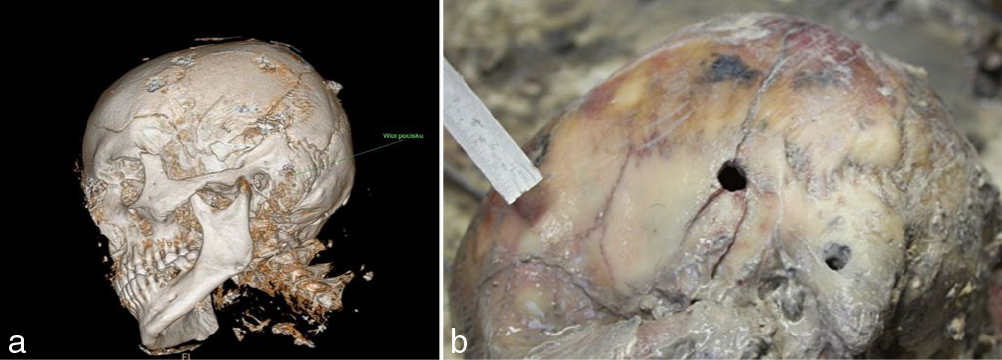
(a) Two entry wounds on the left side of the head seen on post-mortem CT reconstruction. (b) Photograph taken during autopsy.
